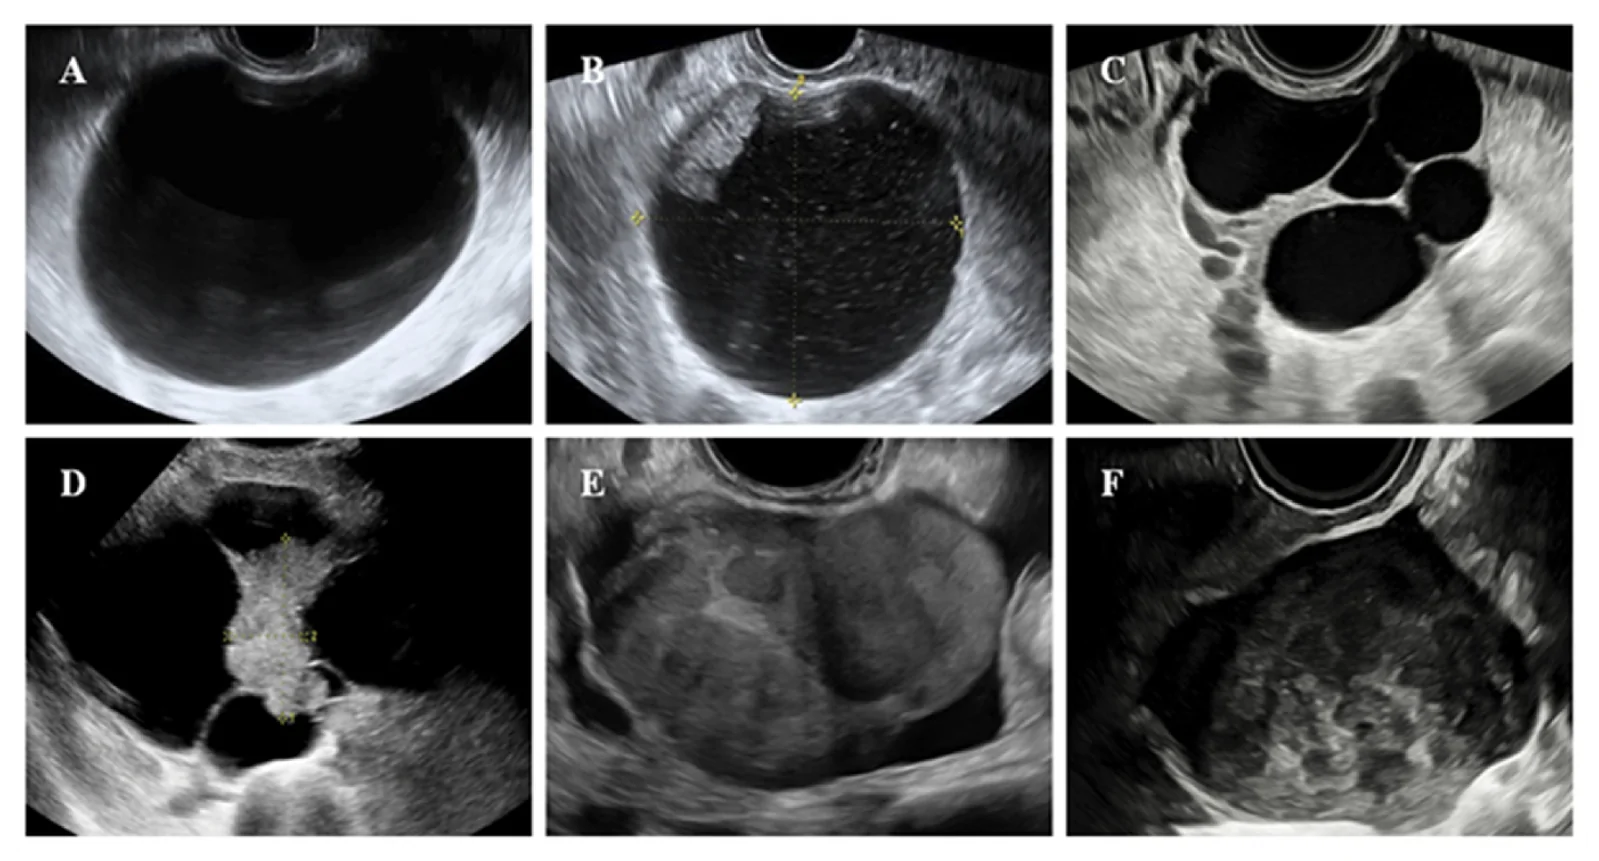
Ultrasound images showing adnexal mass morphologies, according to IOTA terms and definitions: unilocular (A), unilocular solid (B), multilocular (C), multilocular solid (D), solid (E,F).
